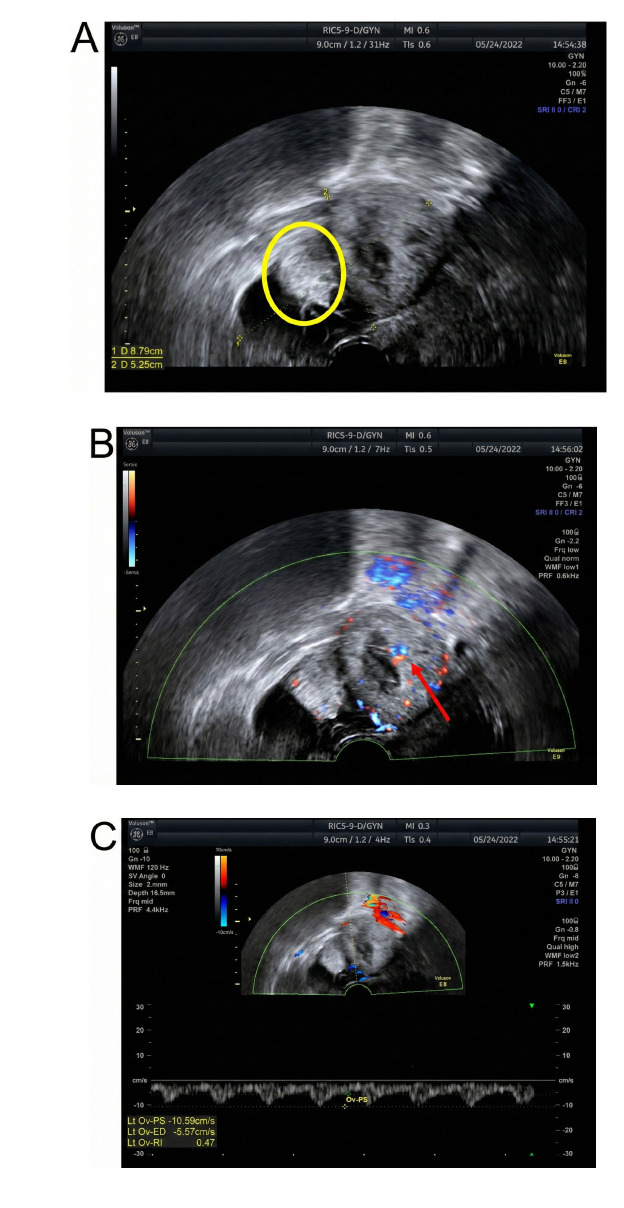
A 55-year-old female patient with struma ovarii. (A) The lesion exhibits clear boundaries, regular morphology, mainly solid mass, and visible calcification (yellow circles). (B) CDFI: The solid part of the lesion shows abundant blood flow signals (red arrow). (C) Color Doppler shows rich blood flow; RI: 0.47.
